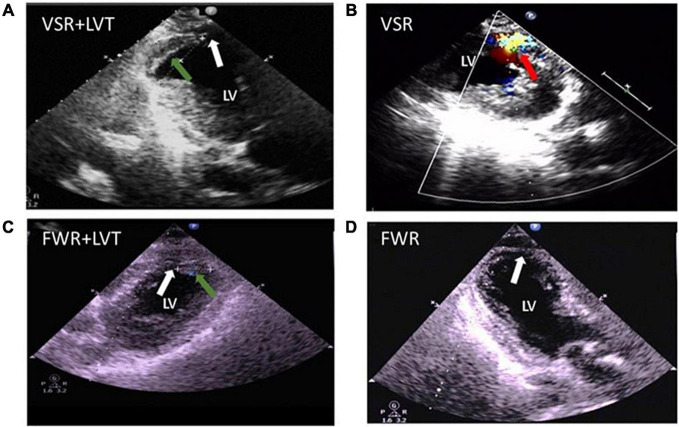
Echocardiographic images from patients complicated with both cardiac rupture (CR) and a left ventricular thrombi (LVT) after acute MI. (A) 2-D image (case 1) showing the presence of LVT (green arrow) and defect of the ventricular septum (VSR, white arrow) at echocardiographic examination (on day 14). (B) Color Doppler image (case 2) obtained on day 5 after MI revealing the *trans*-septum blood shunt indicating ventricular septum rupture (VSR, red arrow). (C,D) 2-D image (case 3 obtained on 5 days) revealed the presence of LVT [green arrow, (C)] and the defect of the apical wall [FWR, white arrow, (D)].
